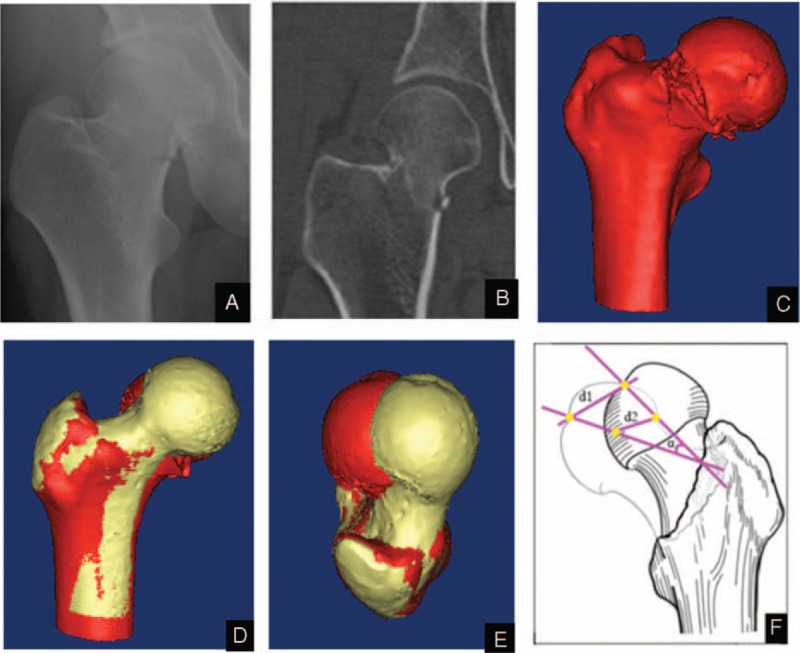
A Garden stage II right femoral neck fracture. (A) Antero-posterior radiograph. (B) Coronal scanning of computed tomography. (C) Three-dimensional model of femoral neck fracture. (D) Front view of bilateral femoral 3-dimensional model. (E) Top views of bilateral femoral 3-dimensional model. The red model represents femoral model with fracture, and the yellow model represents the healthy femoral model. (F) The figures describe the new measuring method for spatial displacements.
